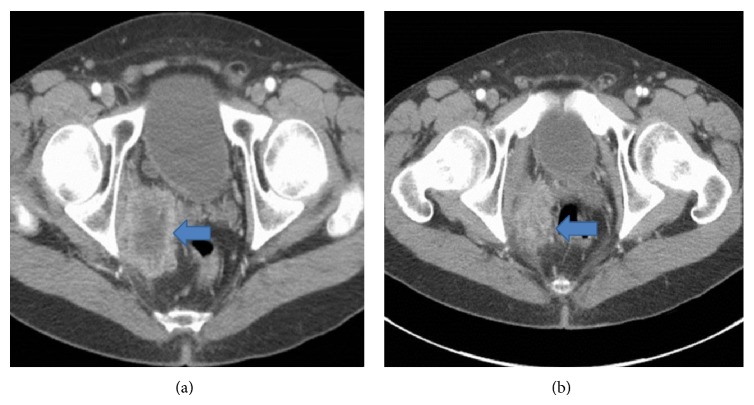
Contrast-enhanced CT scan of the pelvis demonstrates a 6.5 × 5.1 cm mass within the right pelvis (arrows), bordered by the right obturator internus, right seminal vesicles, and bladder diverticulum. The epicenter of the mass is centered at the internal iliac neurovascular bundle.
